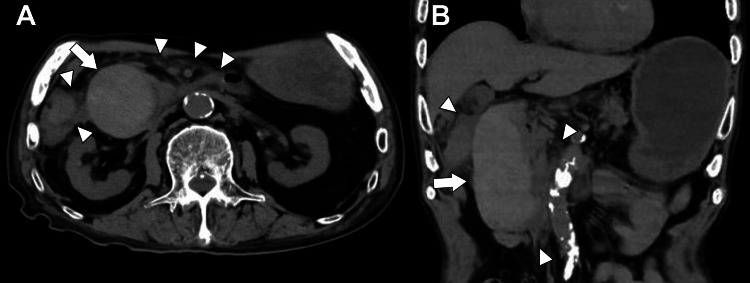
Abdominal computed tomography on day 1 (A) Axial view and (B) coronal view show an intramural hematoma in the descending portion of the duodenum (arrows), with periduodenal and peripancreatic fat stranding and fluid collection (arrowheads).
